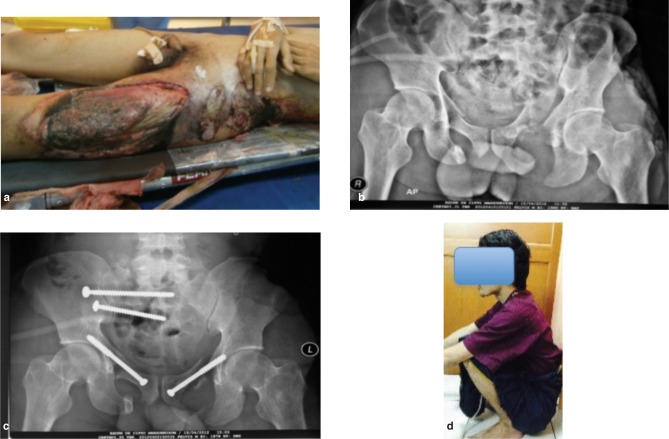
Pelvic fracture MTB 2. (a) clinical picture (b) pre operative radiograph (c) post operative radiograph (d) 2 years after operation with excellent majeed pelvic score.
